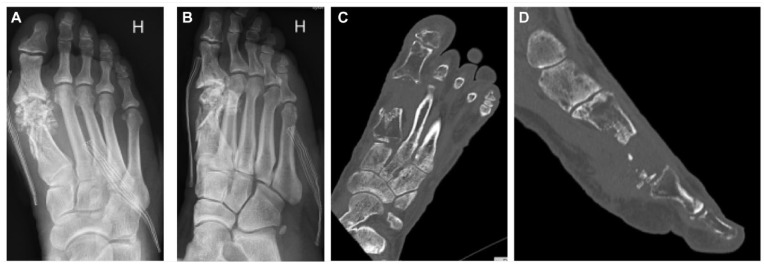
The initial anterioposterior and oblique radiographs show a multifragmented first metatarsal bone, fractured hallux sesamoids and proximal first phalanx (5A and B), and the computed tomography scans show the preoperative state of the injured right foot, with a defect of the first metatarsal bone including the metatarsal head (5C and D).
